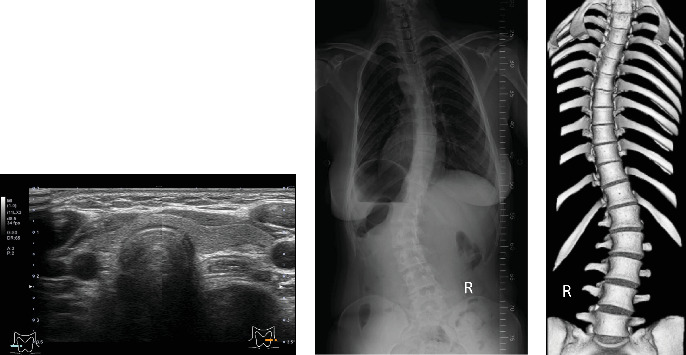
Clinical images of the patient with FHH3. (A) An ultrasonographic image of the neck. (B) The posterior view of the spinal radiograph showing scoliosis. (C) The three-dimensional computed tomography image of the patient. FHH3, Familial hypocalciuric hypercalcemia type 3.
